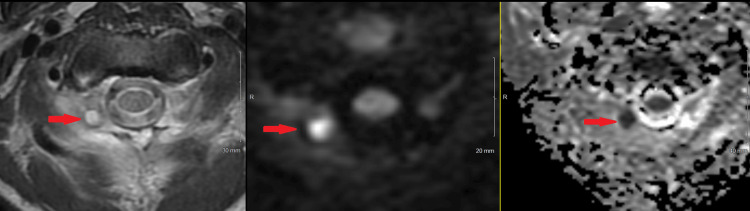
MRI cervical spine axial T2 fast spin echo (left), axial DWI (center), and axial ADC (right) sequences. Findings include a well-circumscribed T2 hyperintense fluid collection involving the right C1-C2 facet joint with diffusion restriction consistent with abscess.
